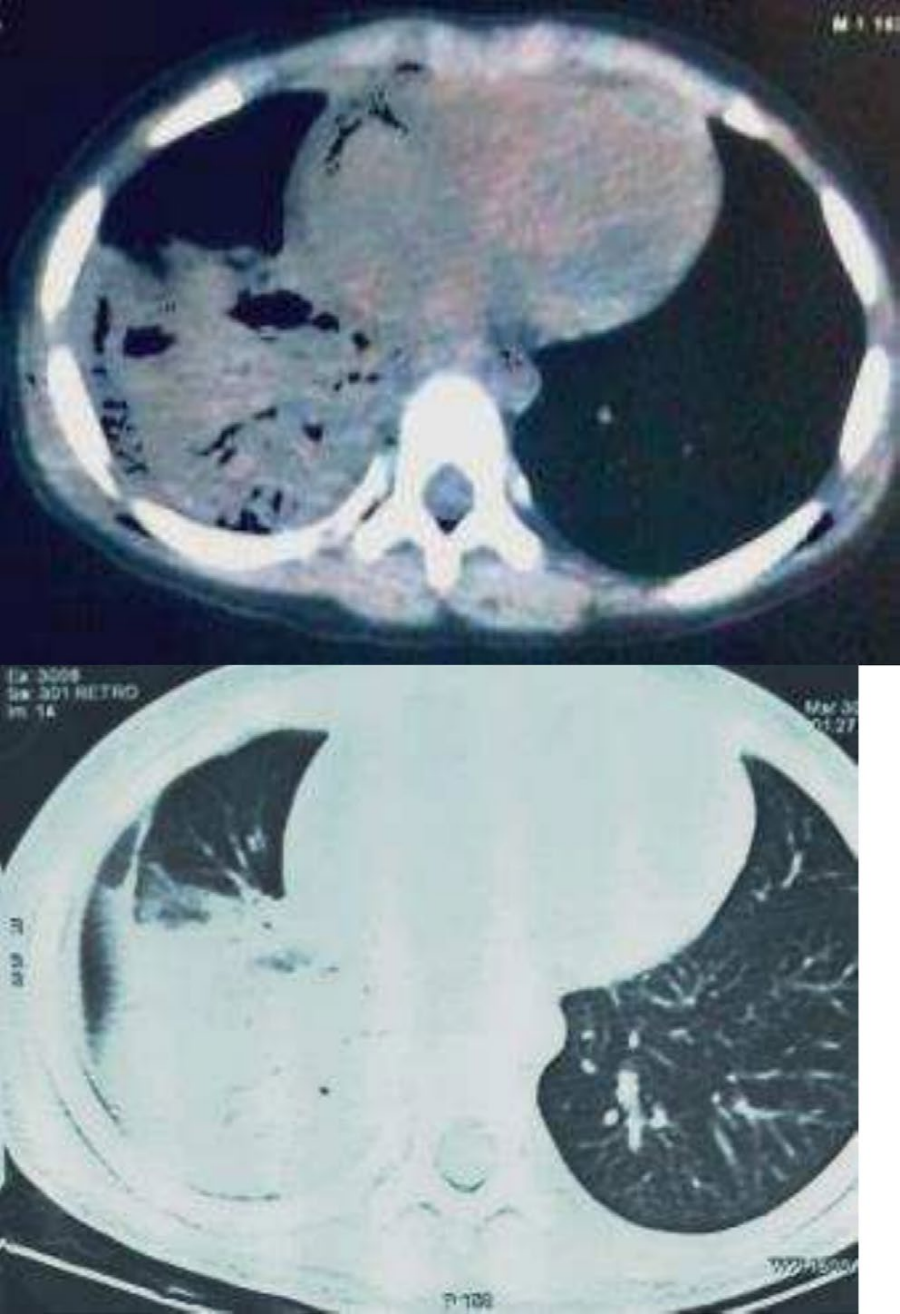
HRCT scan of the chest showing lateral sections of the right middle lobe and the entire right lower lobe showed consolidation with air bronchogram, which was linked to volume loss.
